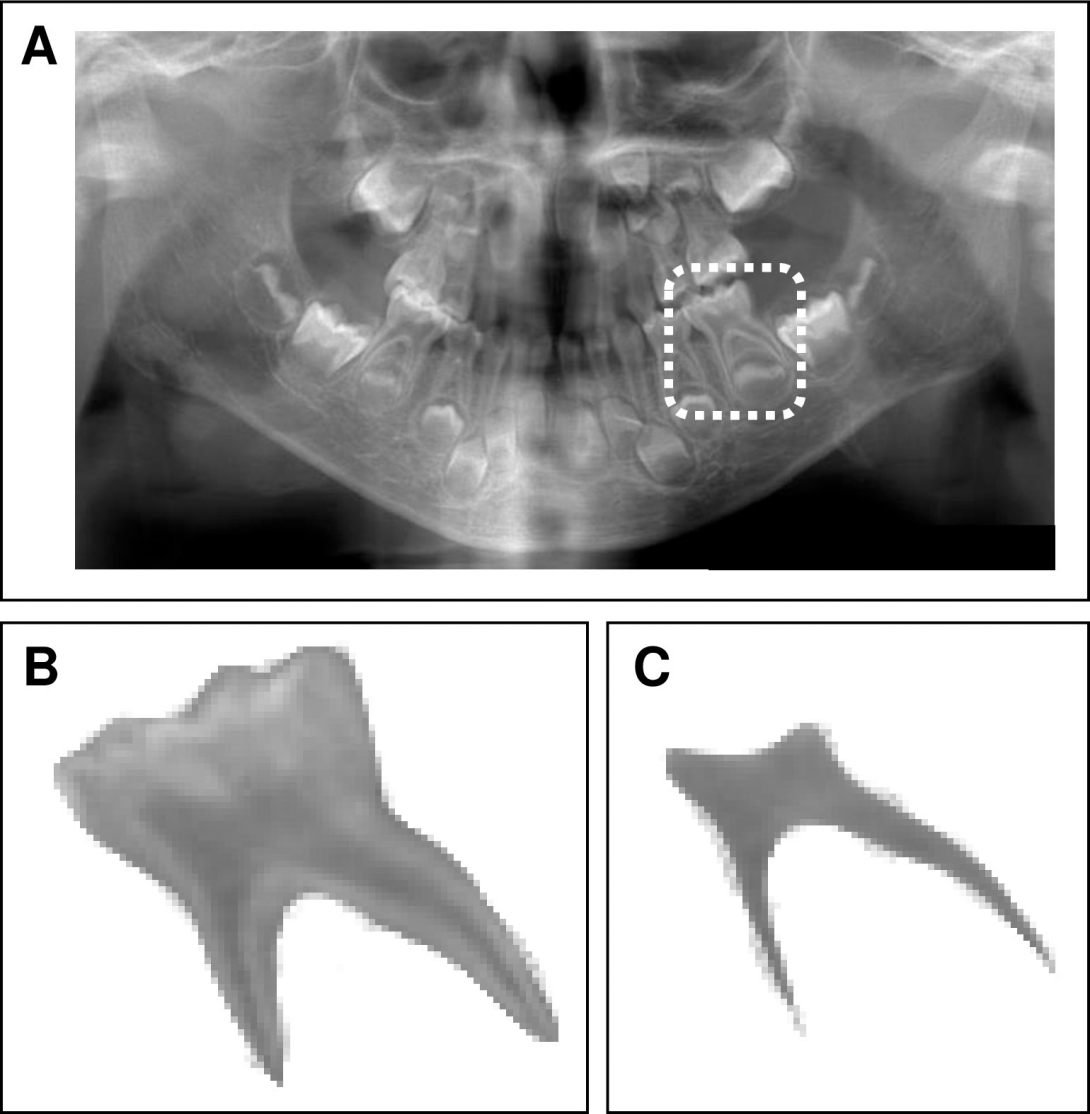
Method for analyzing orthopantomographic images. (A) The target tooth (mandibular left second primary molar) is within the white rectangle. (B) The target tooth trimmed from the orthopantomographic image. (C) The pulp chamber trimmed from the target tooth.
